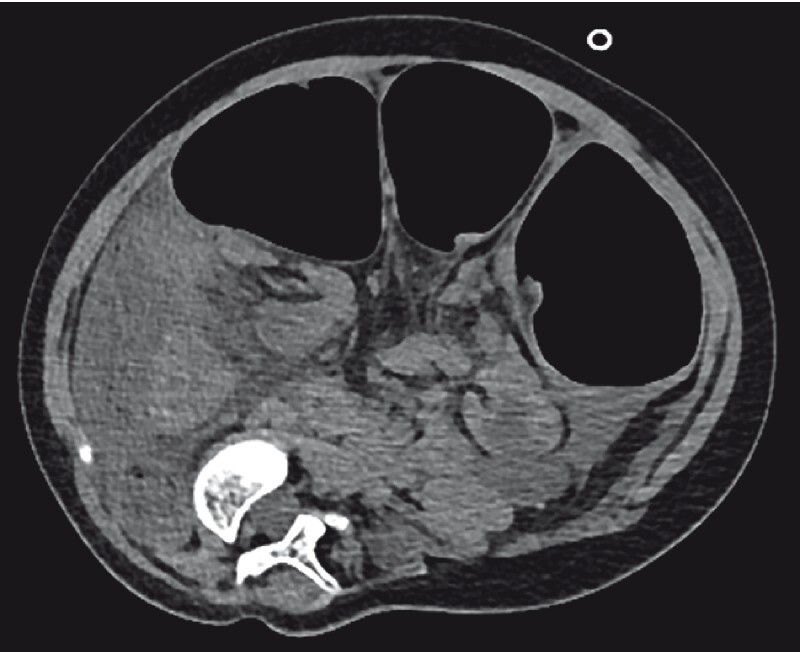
Abdominal computed tomography in the axial plane shows prestenotic dilatation of the sigmoid colon near the point of mesenterial rotation.
